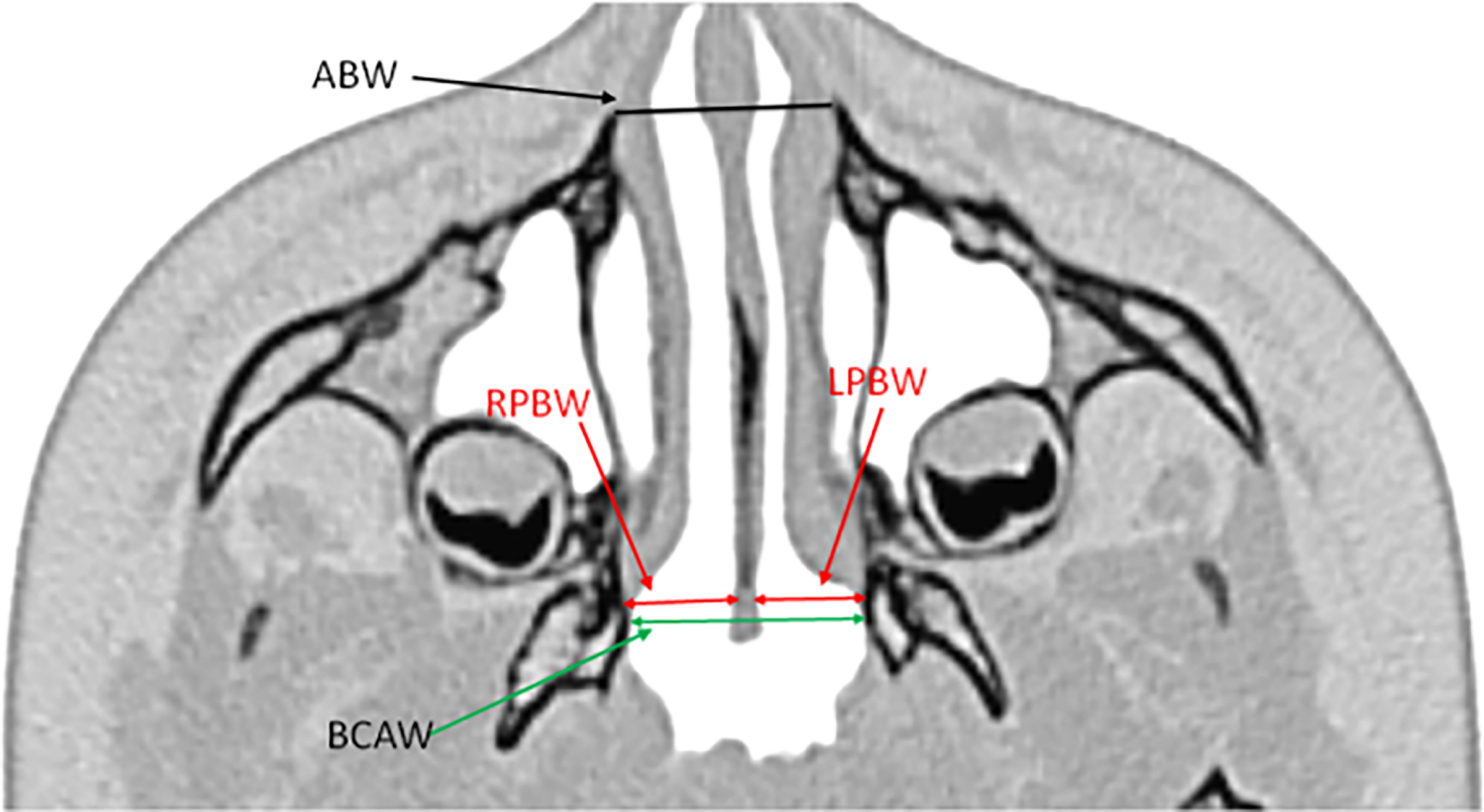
Measurements of nasal cavity dimensions in children with craniosynostosis on CT image, with colour inversion. Abbreviations: ABW—anterior bony width; BCAW—bony choanal aperture width; RPBW—right posterior bony width; LPBW—left posterior bony width.
